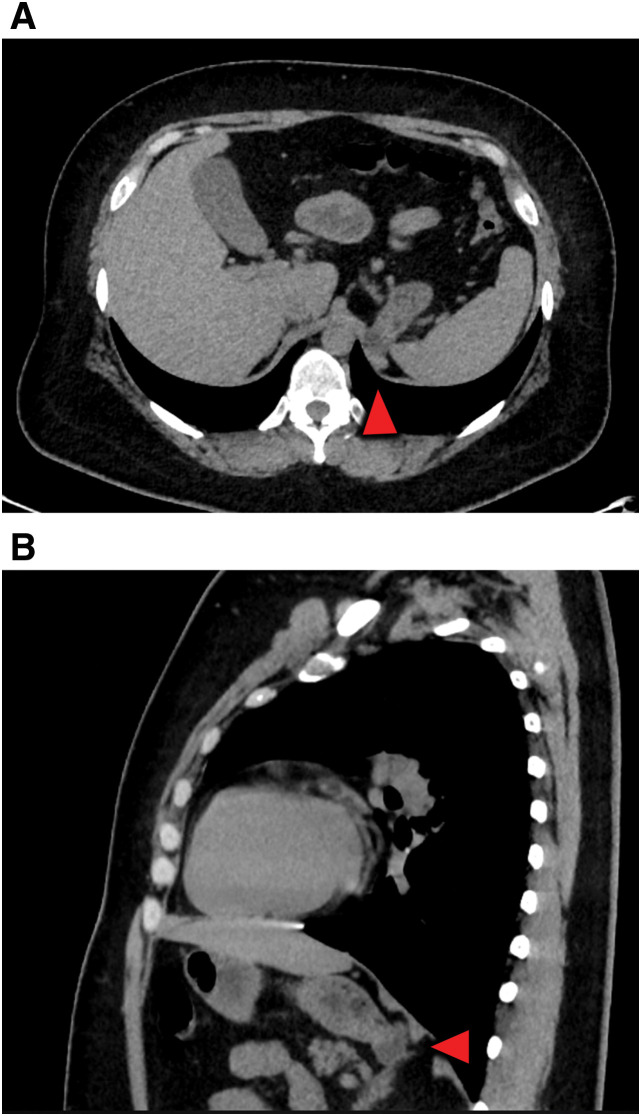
Preoperative plain computed tomography images. A diverticulum protruding dorsally at the fundus of the stomach is observed. The red triangle points to the diverticulum. (A) Axial and (B) sagittal.
